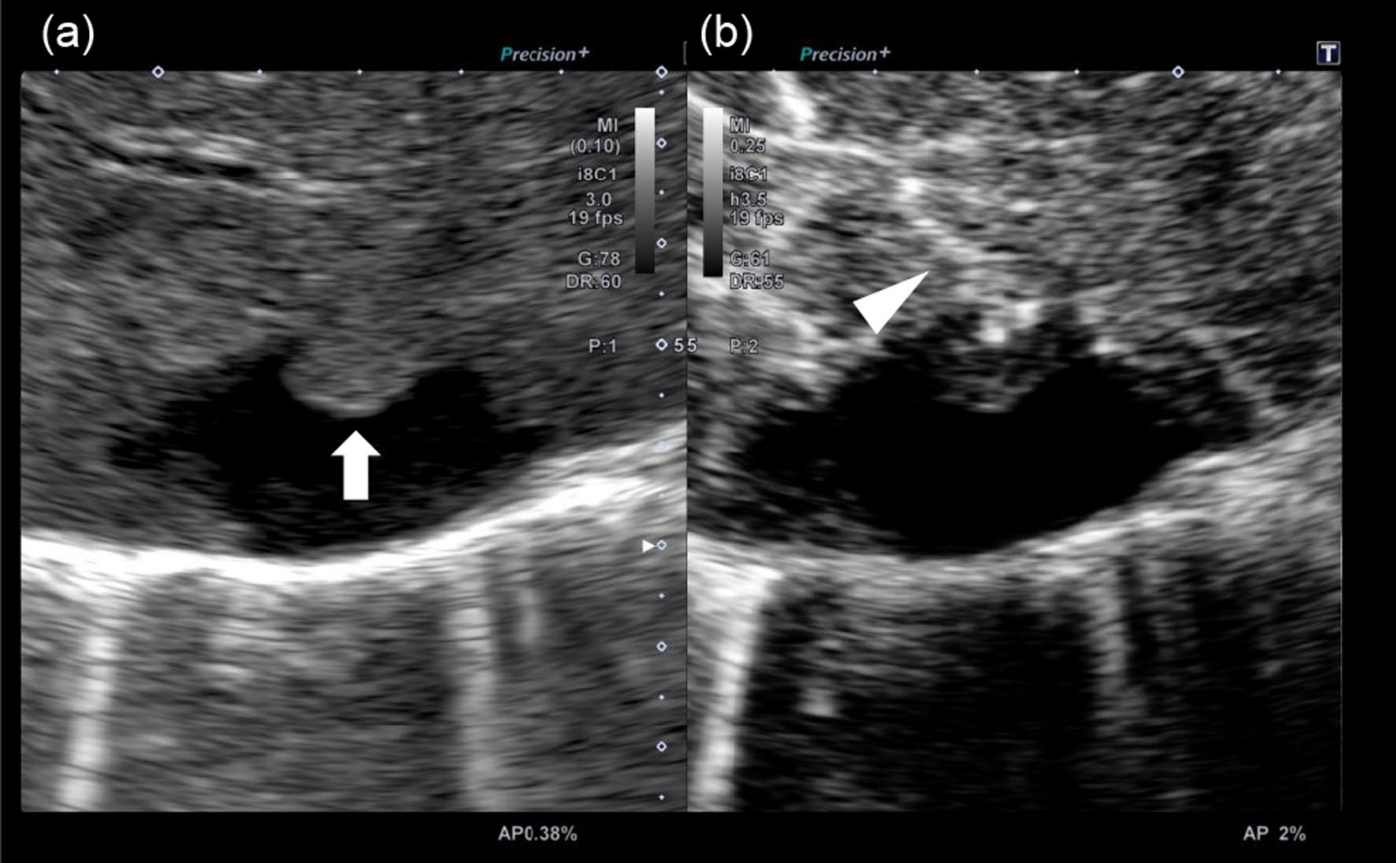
Enhanced ultrasonography. An isoechoic irregular nodule was detected in B-mode ultrasonography (a). A distinct inflow artery is observed toward the nodule in the enhanced ultrasonography (arrowhead) (b)
